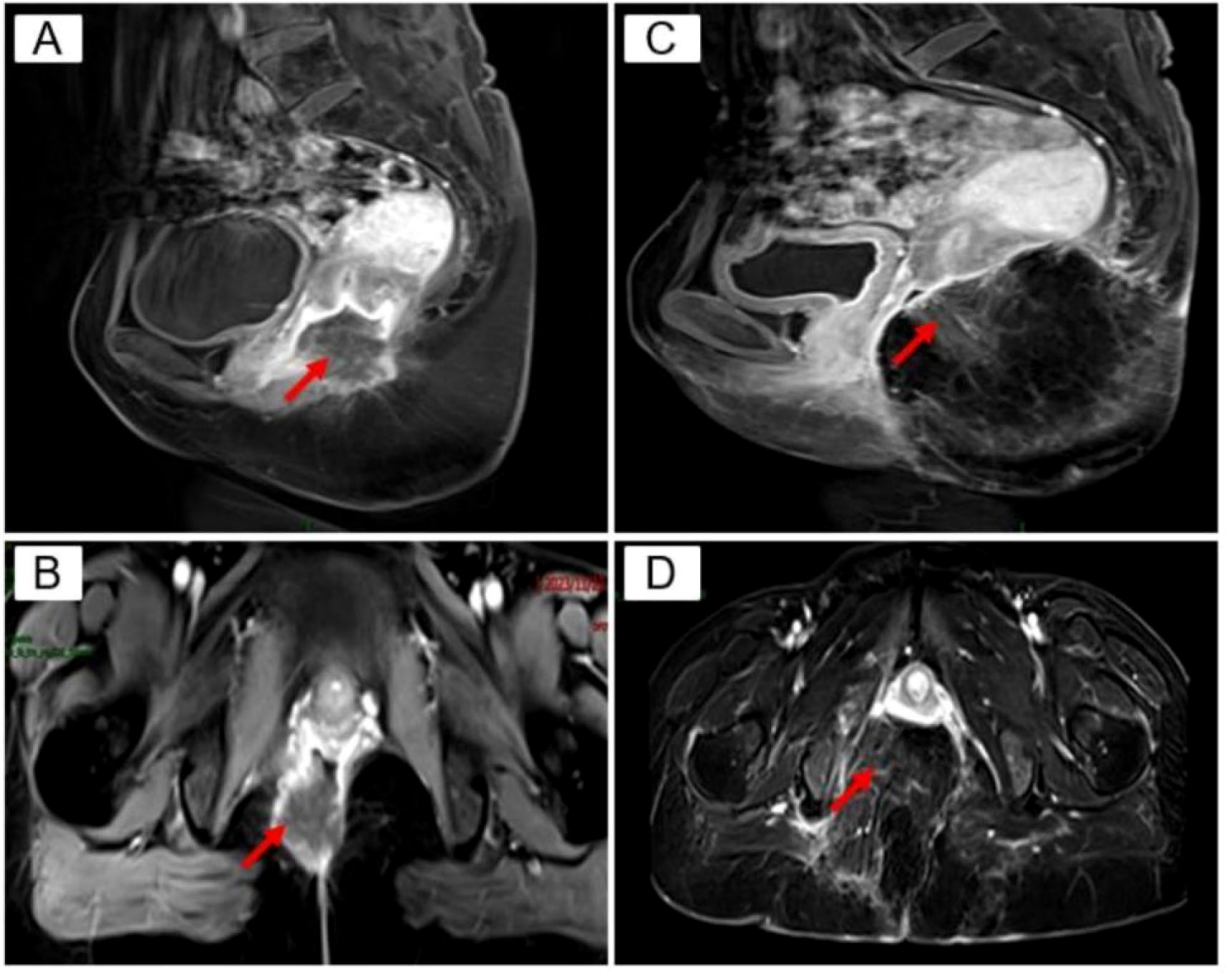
Pelvic MRI. red arrows (A) Preoperative TIWI Cor: annular enhancing mass in the posterior vaginal wall, transverse diameter of about 3.2 3.8 cm, irregular borders, lobulated, seems to see burrs; (B) Preoperative T1 WI Sag: transverse diameter of about 4.0 cm; (C) Postoperative T1W1 Cor: caudal bone is absent, and the mass is normally altered after resection; (D) Postoperative TI WI Sag.
